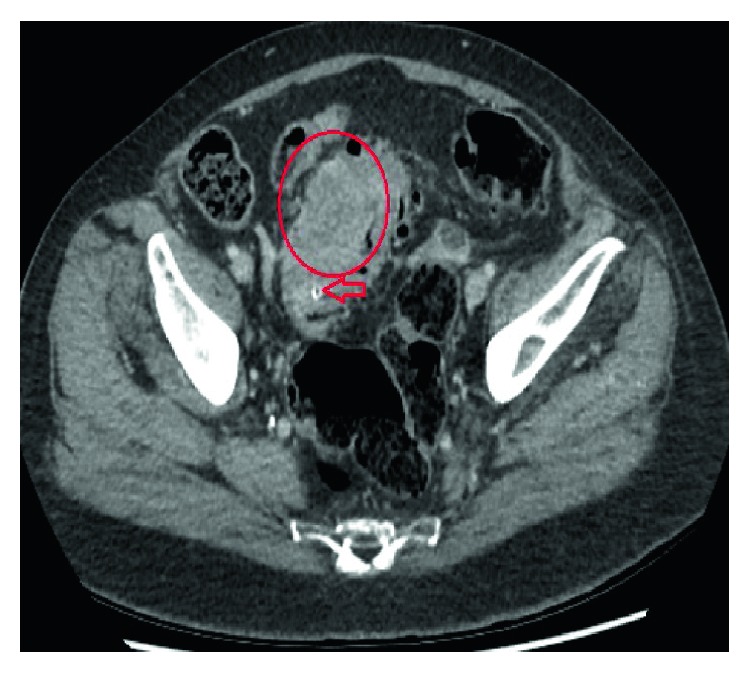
Axial CT scan. Arrow points out a cross-sectional image of the foreign body in question. Meanwhile, the area outlined represents the mass-like thickening of the sigmoid colon proximal to the foreign body.
